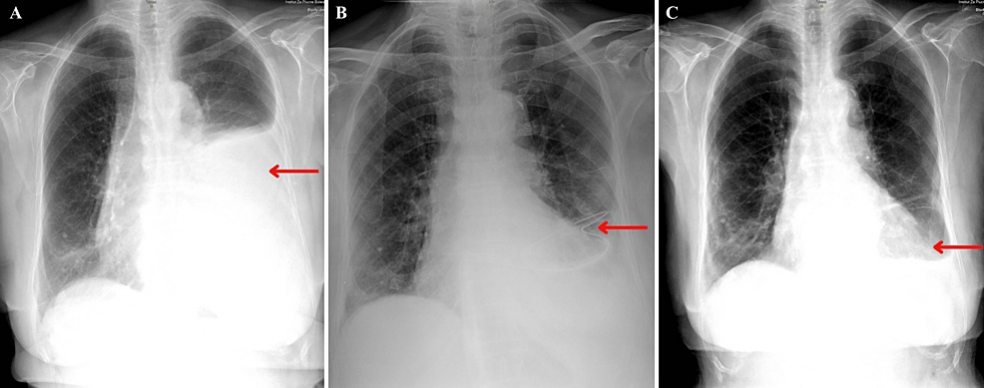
Three anterior-posterior view chest X-rays of the patient with chylothorax (A) Initial chest X-ray; red arrow shows a large, left-sided pleural effusion.
